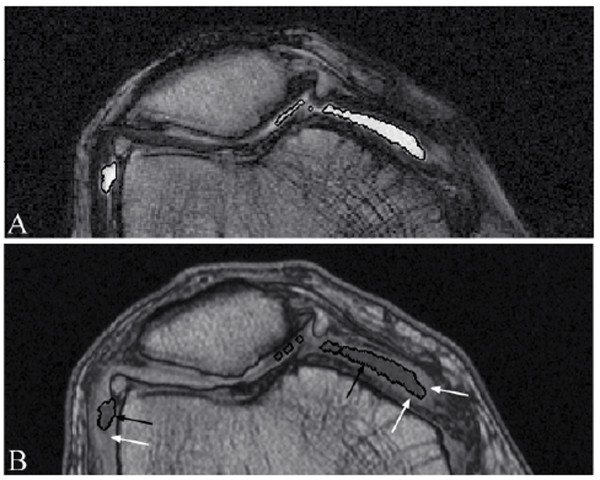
Synovial fluid 3D object presented in both T1 and T2 MR images. (A) Synovial effusion segmentation (white contours) of a representative knee osteoarthritis patient MRI T2 image slice and (B) transported into the corresponding T1 image (black contours), showing that the synovial membrane (bright tissue; white arrows) is excluded from the joint effusion objects (black arrows).
